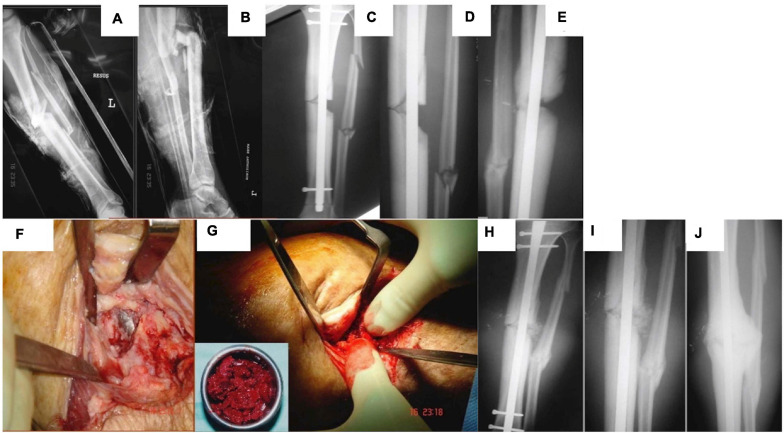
A male patient 29 years of age sustained a left tibial open fracture. (A) Initial anterior–posterior (AP). (B) Lateral radiographs of left tibia showing the midshaft fracture. (C) AP. (D) Lateral tibial radiographs showing that the fracture was stabilized with an intramedullary nail (note the tibial small defect laterally). (E) Lateral tibial radiograph at 4 months demonstrating lack of healing activity. (F) Intraoperative picture at 6 months showing non-union/bone loss and visualization of the nail. (G) Intraoperative picture showing bone grafting of the tibia with autologous bone graft. (H) AP postoperative. (I) Closer AP postoperative radiograph showing the area of small bone defect filled with the autologous bone graft. (J) AP radiograph 3 months lateral showing osseous healing of the tibial small defect.
